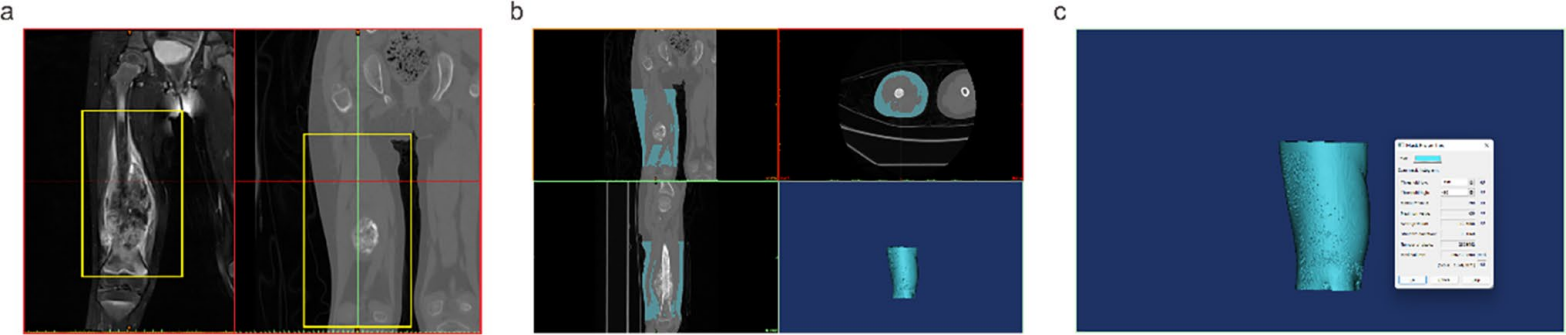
a Map of the OS region (yellow frame) on the MRI image to the CT image. b Establish the mask and the 3D anatomic model of the peri-osteosarcoma adipose. c The average CT attenuation and the total volume of the peri-osteosarcoma were displayed in the mask properties windows
